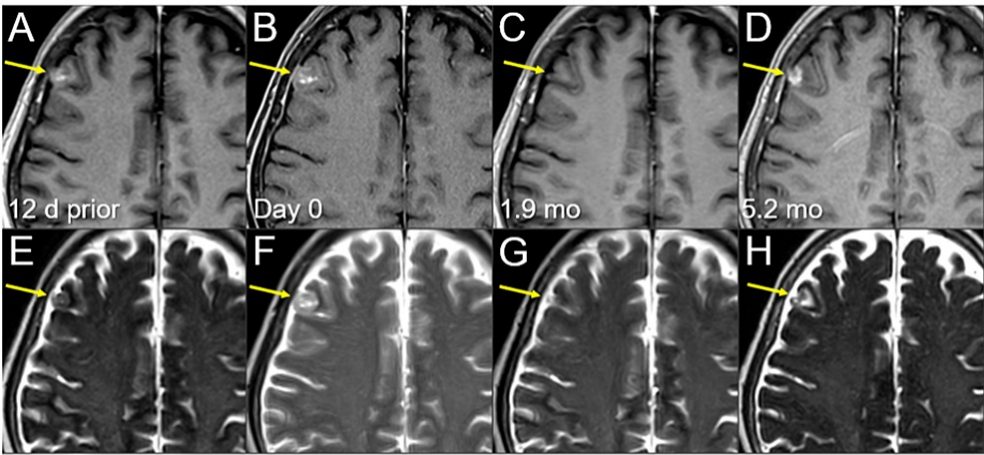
Magnetic resonance images before and after the second single-fraction radiosurgery of the right frontal lesion. The images show (A-D) axial CE-T1-WIs and (E-H) axial T2-WIs (A, E) 12 days before (12 d prior) the second SRS (11.8 months after the initiation of CRT); (B, F) on the day of SRS (day 0); (C, G) at 1.9 mos after the second SRS; and (D, H) at 5.2 months (17.3 months after CRT). (A-H) These images are shown at the same magnification and coordinates under co-registration and fusions. (A, E) A heterogeneously enhancing lesion (arrow in A) without perilesional edema (arrow in E). (B, F) The lesion increased in size with higher intensity on T2-WIs (arrows in E and F) in 12 days, and the contrast enhancement is faint and partial (arrow in B) compared to the visible mass on  T2-WI (T2 mass, arrow in F), a paradoxical T1/T2 mismatch. Furthermore, the main location of the lesion is the brain surface, the cerebral cortex, with partly exophytic growth beyond the brain surface and attachment to the adjacent dura mater. (C, G) At 1.9 mos, the lesion shrank remarkably (arrows in C and G). (D, H) The enhancing lesion and T2 mass obviously increased (arrows in D and H), along with the development of perilesional edema. CE, contrast-enhanced; WIs, weighted images; SRS, stereotactic radiosurgery; CRT, chemoradiotherapy; T2 mass, a visible mass on T2-WIs; mo, month
